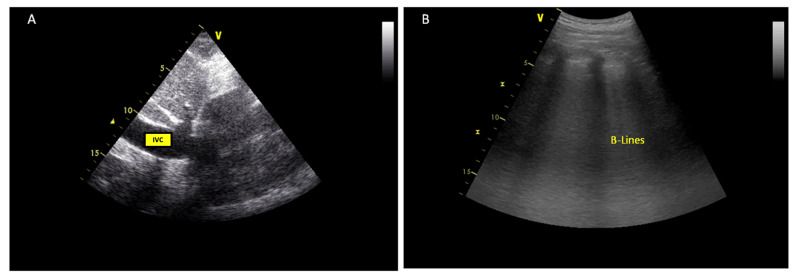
Echocardiographic markers of congestion. (A) increased IVC inferior vena cava (IVC) diameter; (B) lung ultrasound showing vertical B-lines as a measure of interstitial oedema.
